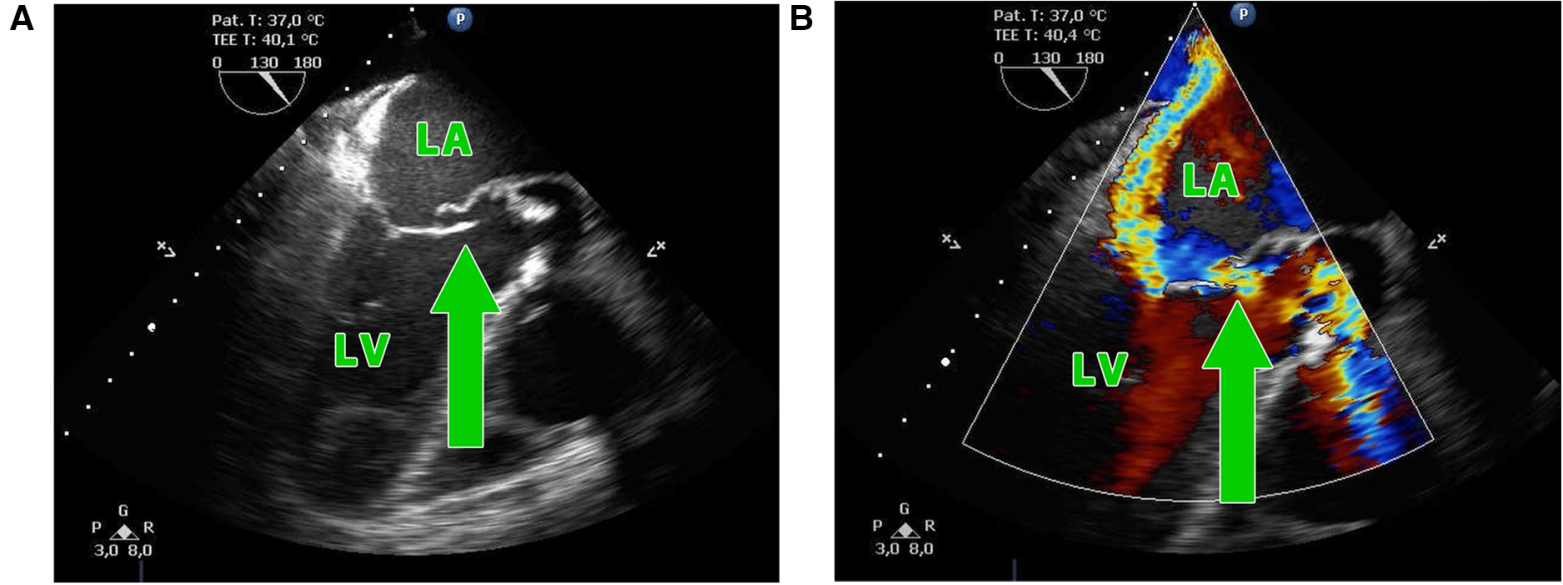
(A,B) Transesophageal echocardiography showing the perforation of the aorto-mitral curtain (green arrow) in a patient with double-valve endocarditis; LA, left atrium; LV, left ventricle.
